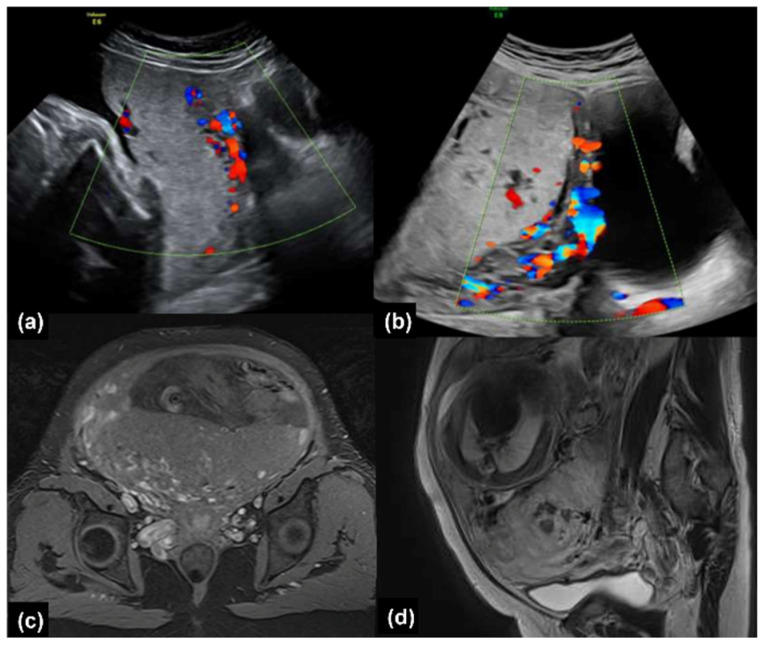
(a) Color Doppler ultrasound examination of abnormal placental lacunae and uterovesical hypervascularity (Case 1), and (b) abnormal placental lacunae, placenta bulging and uterovesical hypervascularity (Case 2). (c) T1-weighted transverse magnetic resonance images (MRI) demonstrating prominent vascularity around the uterus (Case 1). (d) T1-weighted sagittal MRI demonstrating low signal intensity area in the placenta (Case 2).
